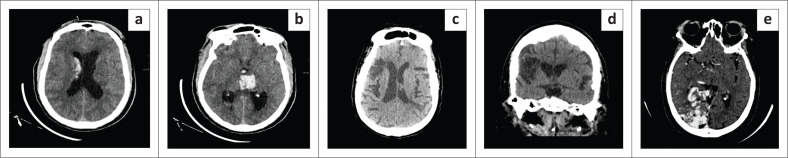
Vascular pathology as a cause of adult-onset seizures. (a and b) Hypertensive intraparenchymal haemorrhage in a 56-year-old male with newly diagnosed hypertension. Axial non-enhanced CT (NECT): Left thalamic haemorrhage with minimal surrounding oedema. Associated intraventricular blood. (c and d) Chronic right middle cerebral artery territory infarction in an adult patient with index episode of seizures. Axial and coronal NECT: Right MCA territory wedge-shaped hypodensity with mild right lateral ventricular ex vacuo dilatation. (e) Right occipital lobe arteriovenous malformation in a 53-year-old female with no background medical history. Axial contrast enhanced CT (CECT): ‘Bag of worms’ appearance of draining and feeding vessels in the right occipital lobe.
